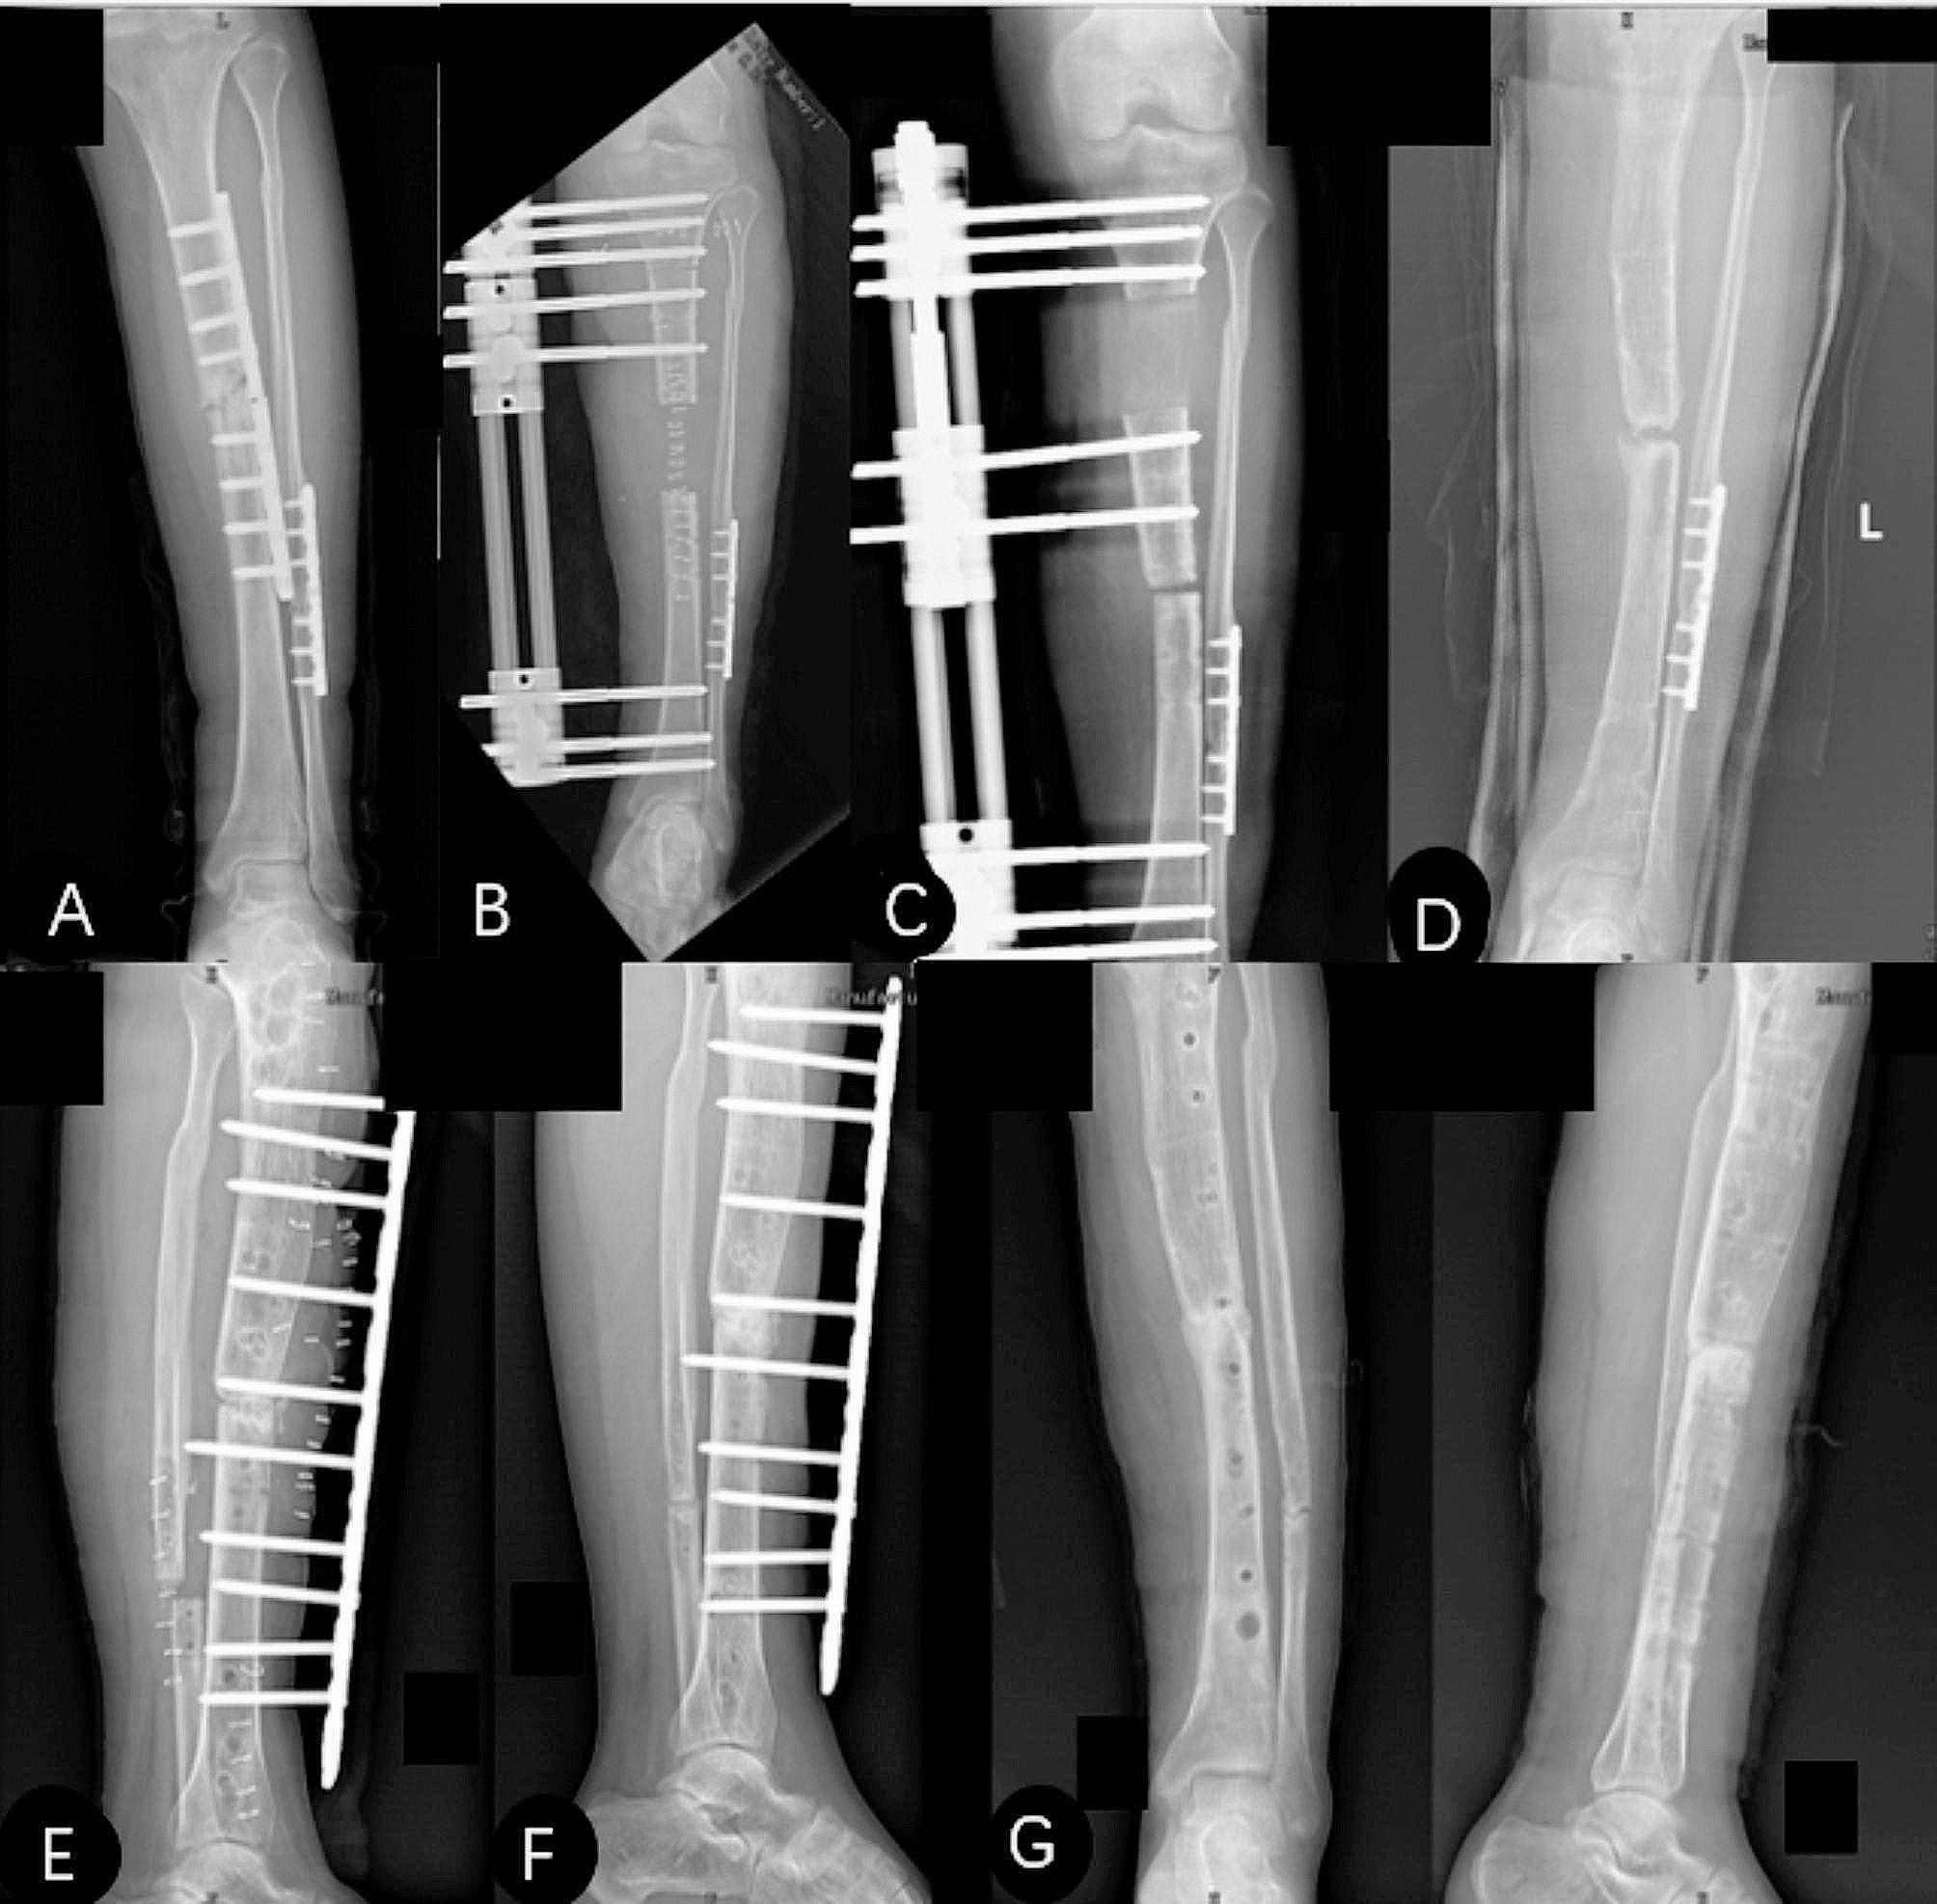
A 47-year-old female present with post-traumatic osteomyelitis of the left tibia underwent Bifocal bone transport using the Ilizarov technique. Docking site refracture was encountered 4.2 months after the removal of the external fixator, which was managed by percutaneous plate external fixation along with autologous bone grafting. (A) Anteroposterior radiograph showed plate refracture and fracture-related infection after open reduction and internal fixation. (B) Anteroposterior radiograph on the first postoperative day after application of bifocal bone transport using the Ilizarov technique. (C) Anteroposterior radiograph during bone transport. (D) The docking site refracture was encountered 4.2 months after the removal of the fixator and was managed temporarily by casting. (E) Anteroposterior radiograph demonstrated docking site refracture managed by percutaneous plate external fixation along with autologous bone grafting. (F) Anteroposterior radiographs at 6.8 months follow-up showed union at the docking site. (G) Anteroposterior radiograph at last clinical visit after removal of percutaneous plate external fixation
